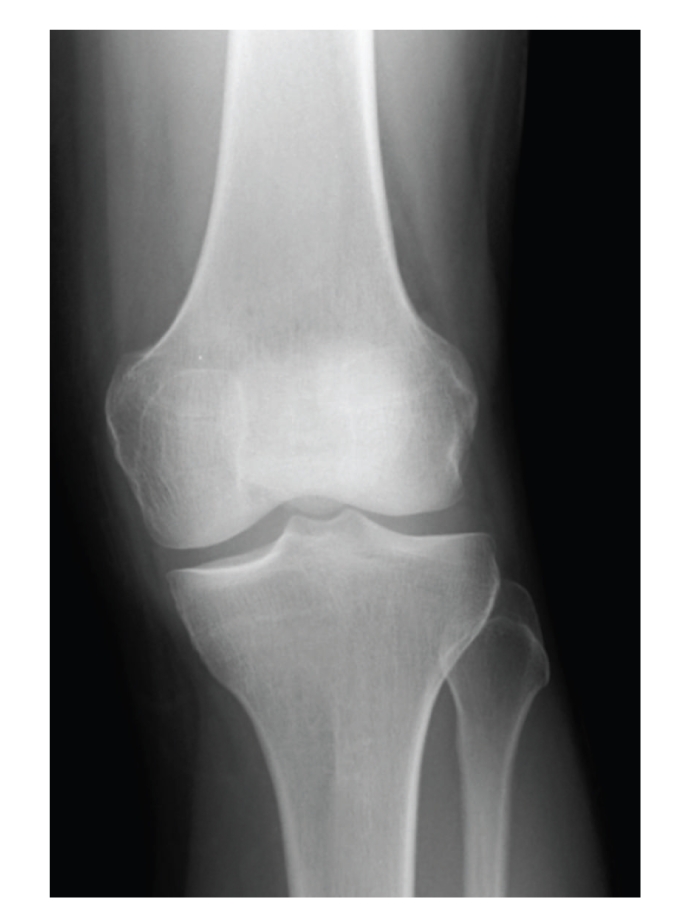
Normal AP radiograph of left knee.
